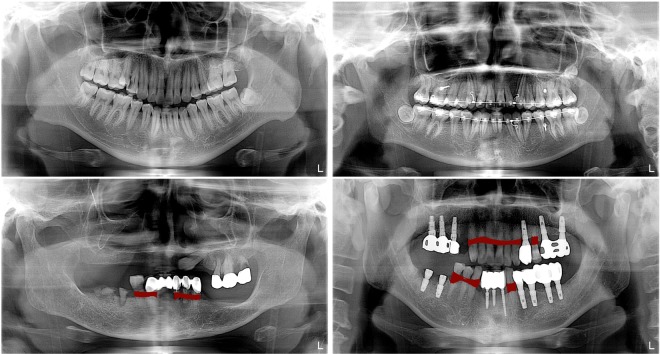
Sample panoramic dental radiographs and annotated PBL lesions. (Top) Normal cases without annotated periodontal bone loss lesion, (Bottom) Abnormal cases with annotated lesion masks. The annotators also provided corresponding teeth numbers of the PBL lesions.
